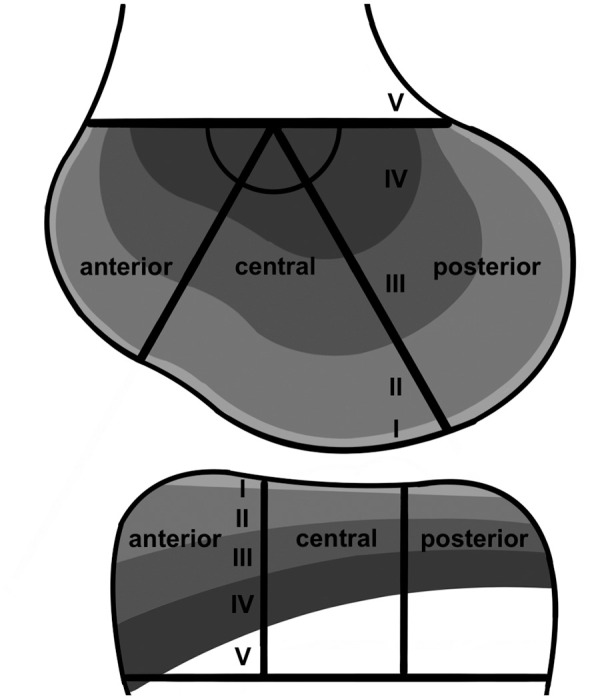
Segmentation technique according to the modified WORMS (Whole-Organ MRI Score) method.^6,37^ A horizontal line was drawn along the physeal scar. Starting from the midpoint of the femoral line, 2 lines, each at an angle of 60°, defined the borders of the anterior, central, and posterior femoral segments. At the tibia, the line was divided into anterior, central, and posterior thirds by vertical lines. Bone bruise depth was classified as superficial if it was below the subchondral bone (I), shallow if it was up to one-third of the distance to the physeal scar (II), deep if it was between one-third and two-thirds of the distance to the physeal scar (III), extensive if it was at least two-thirds of the distance to the physeal scar but did not extend beyond it (IV), or generalized if it extended beyond the physeal scar (V).^
